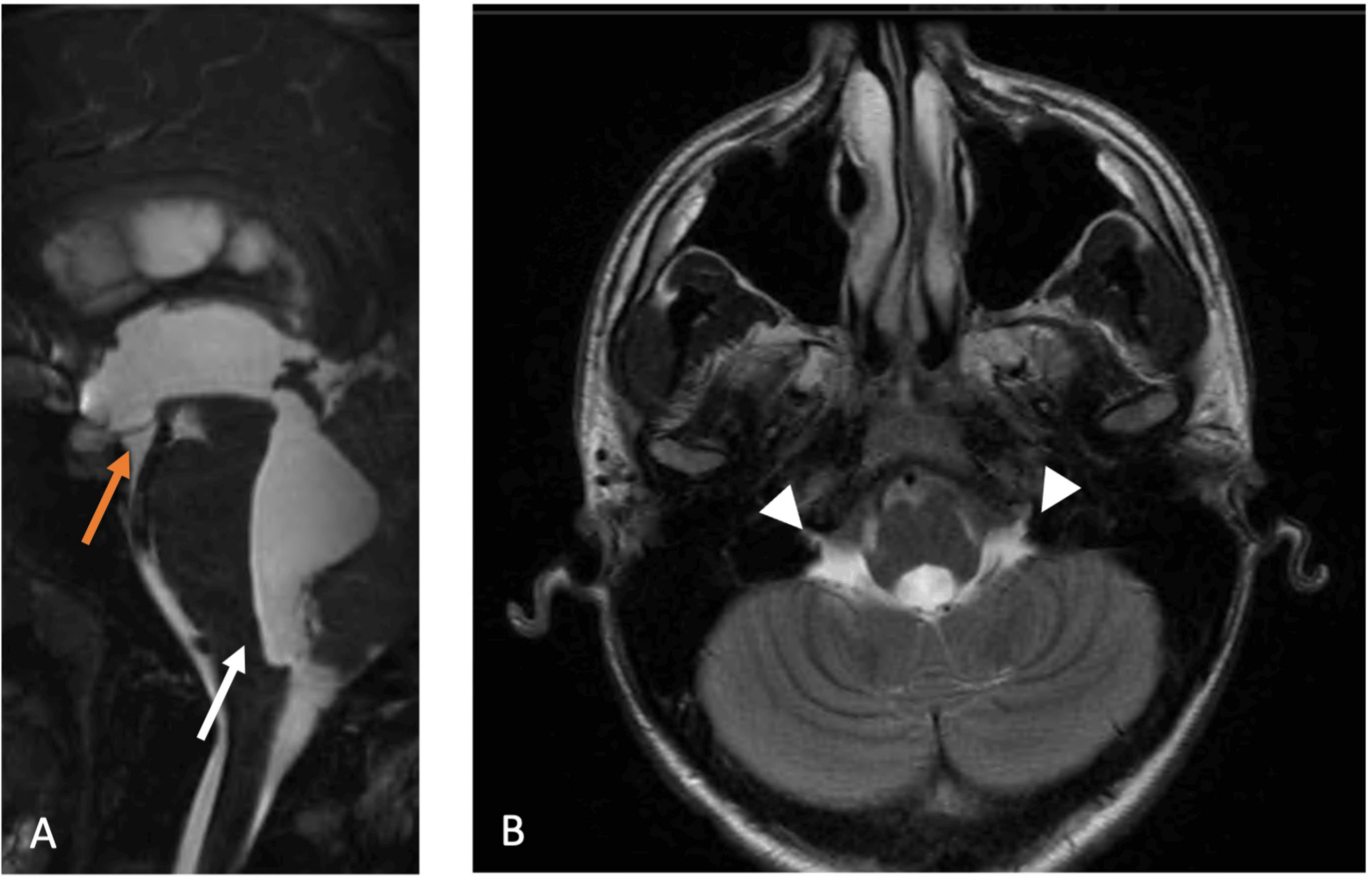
MRI from 2011 with T2-weighted submillimetre volumetric high-resolution imaging showing no clear membrane. A: bowing of the medulla (white arrow) and a low position of the floor of the third ventricle (orange arrow). B: Luschka foramina slightly enlarged (arrowheads).
